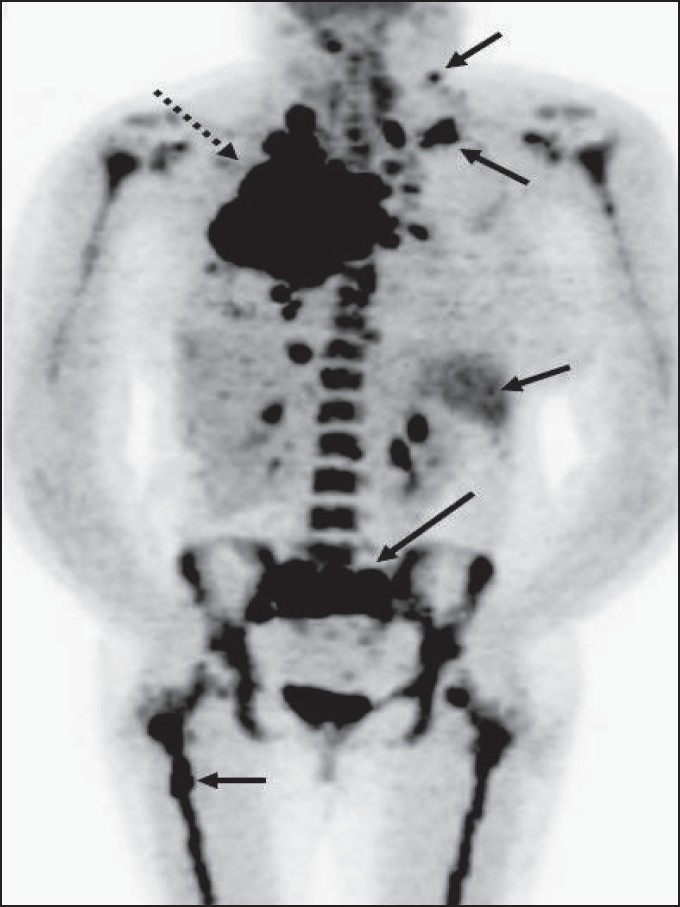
MIP image from a PET study of a 35-year-old lady presenting with a large FDG-avid right lung mass (dotted arrow). The widespread neoplastic disease, along with increased splenic and bone marrow uptake (arrows), suggests lymphoma as the possible underlying etiology. The patient was proven to have HD
